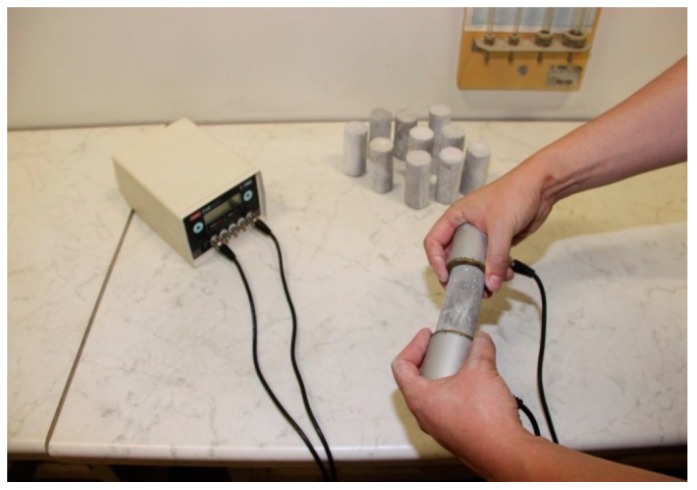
Conduction of ultrasound testing on grout samples.
